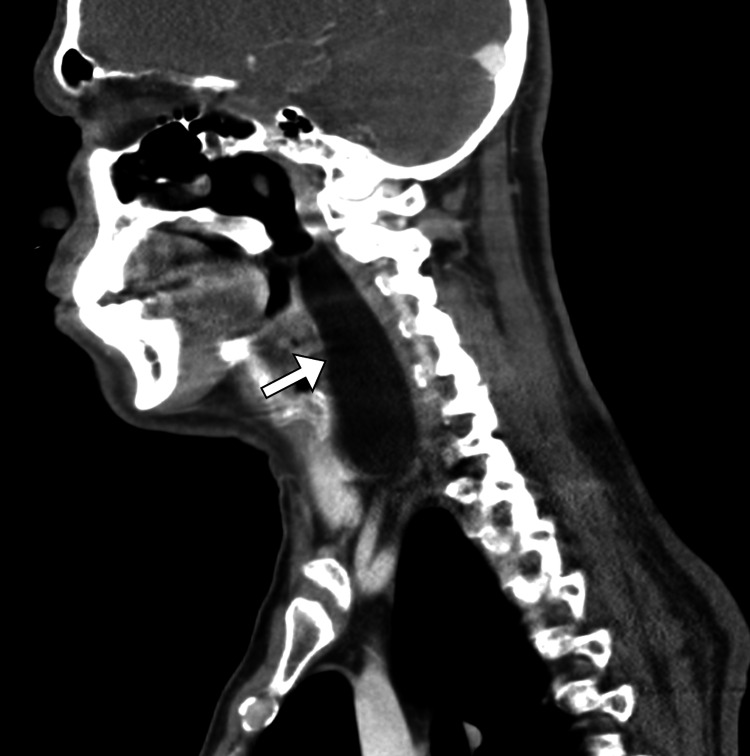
Sagittal CT image of the neck showing a well-defined retropharyngeal lesion with fat density (arrow). CT: computed tomography.
